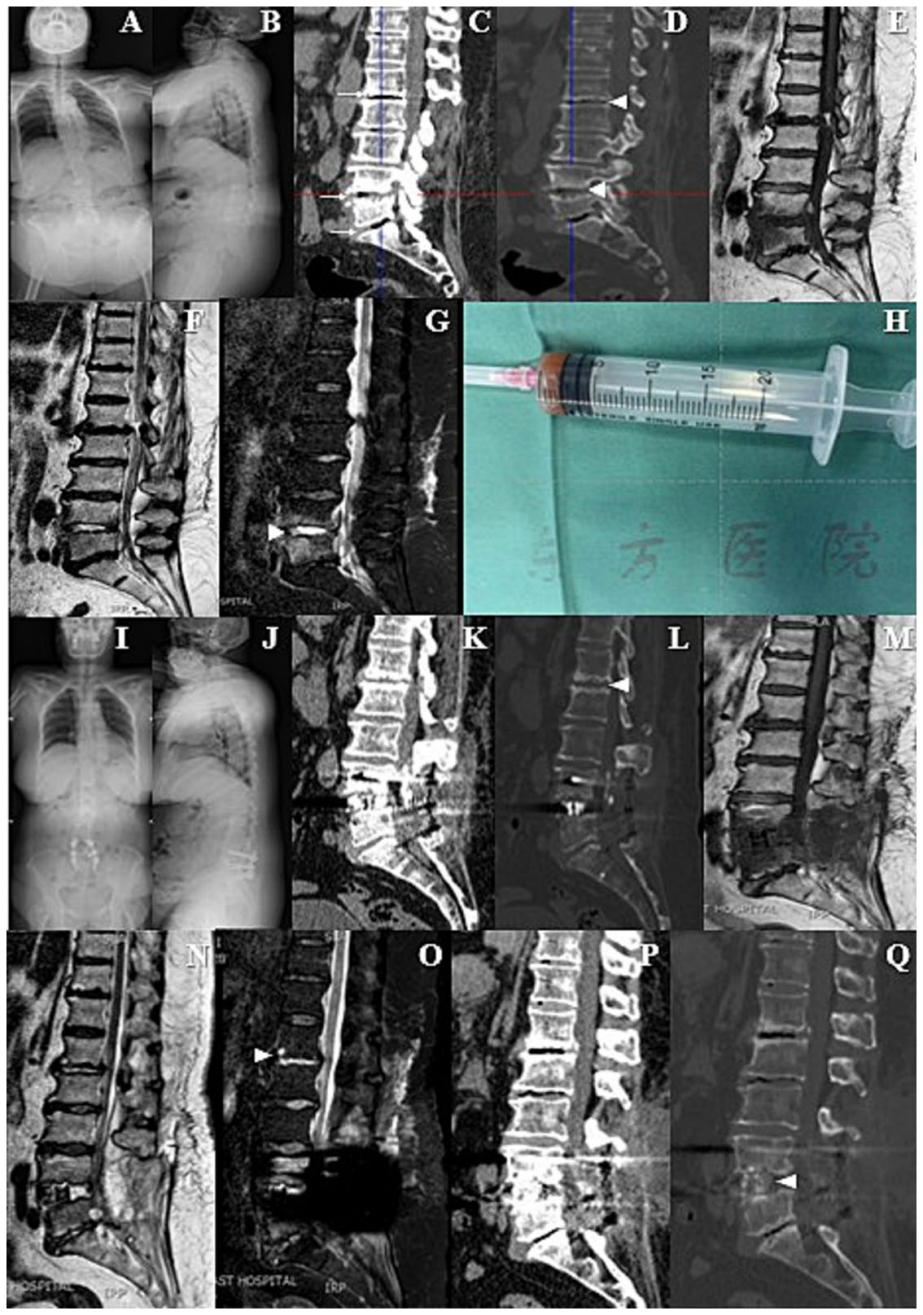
(A,B) Preoperative X-ray films showed scoliosis and degenerative changes in the spine. (C,D) Preoperative computed tomography (CT) revealed obvious linear gas density shadows in the L1/2, L4/5 and L5/S1 intervertebral discs. In addition, the upper and lower endplates of L1/2 and L4/5 vertebrae showed characteristic “worm-eaten” destructive changes. (E–G) Preoperative magnetic resonance imaging (MRI) demonstrated significantly enhanced signals in the L4/5 intervertebral disc. (H) During the operation, obvious destruction of the L4/5 intervertebral disc was observed, accompanied by reddish-brown fluid exudation, which was aspirated for subsequent bacterial culture, fungal culture and gene sequencing. (I–L) Pre-discharge X-ray and CT films indicated thorough decompression and satisfactory internal fixation. (M–O) Follow-up MRI showed that the high signal intensity of L4/5 intervertebral disc had decreased, while the signal brightness of L1/2 intervertebral disc had increased. (P,Q) Follow-up CT showed no significant difference compared with the pre-discharge CT, except for new bone formation in the L4/5 intervertebral space that did not meet the fusion criteria.
